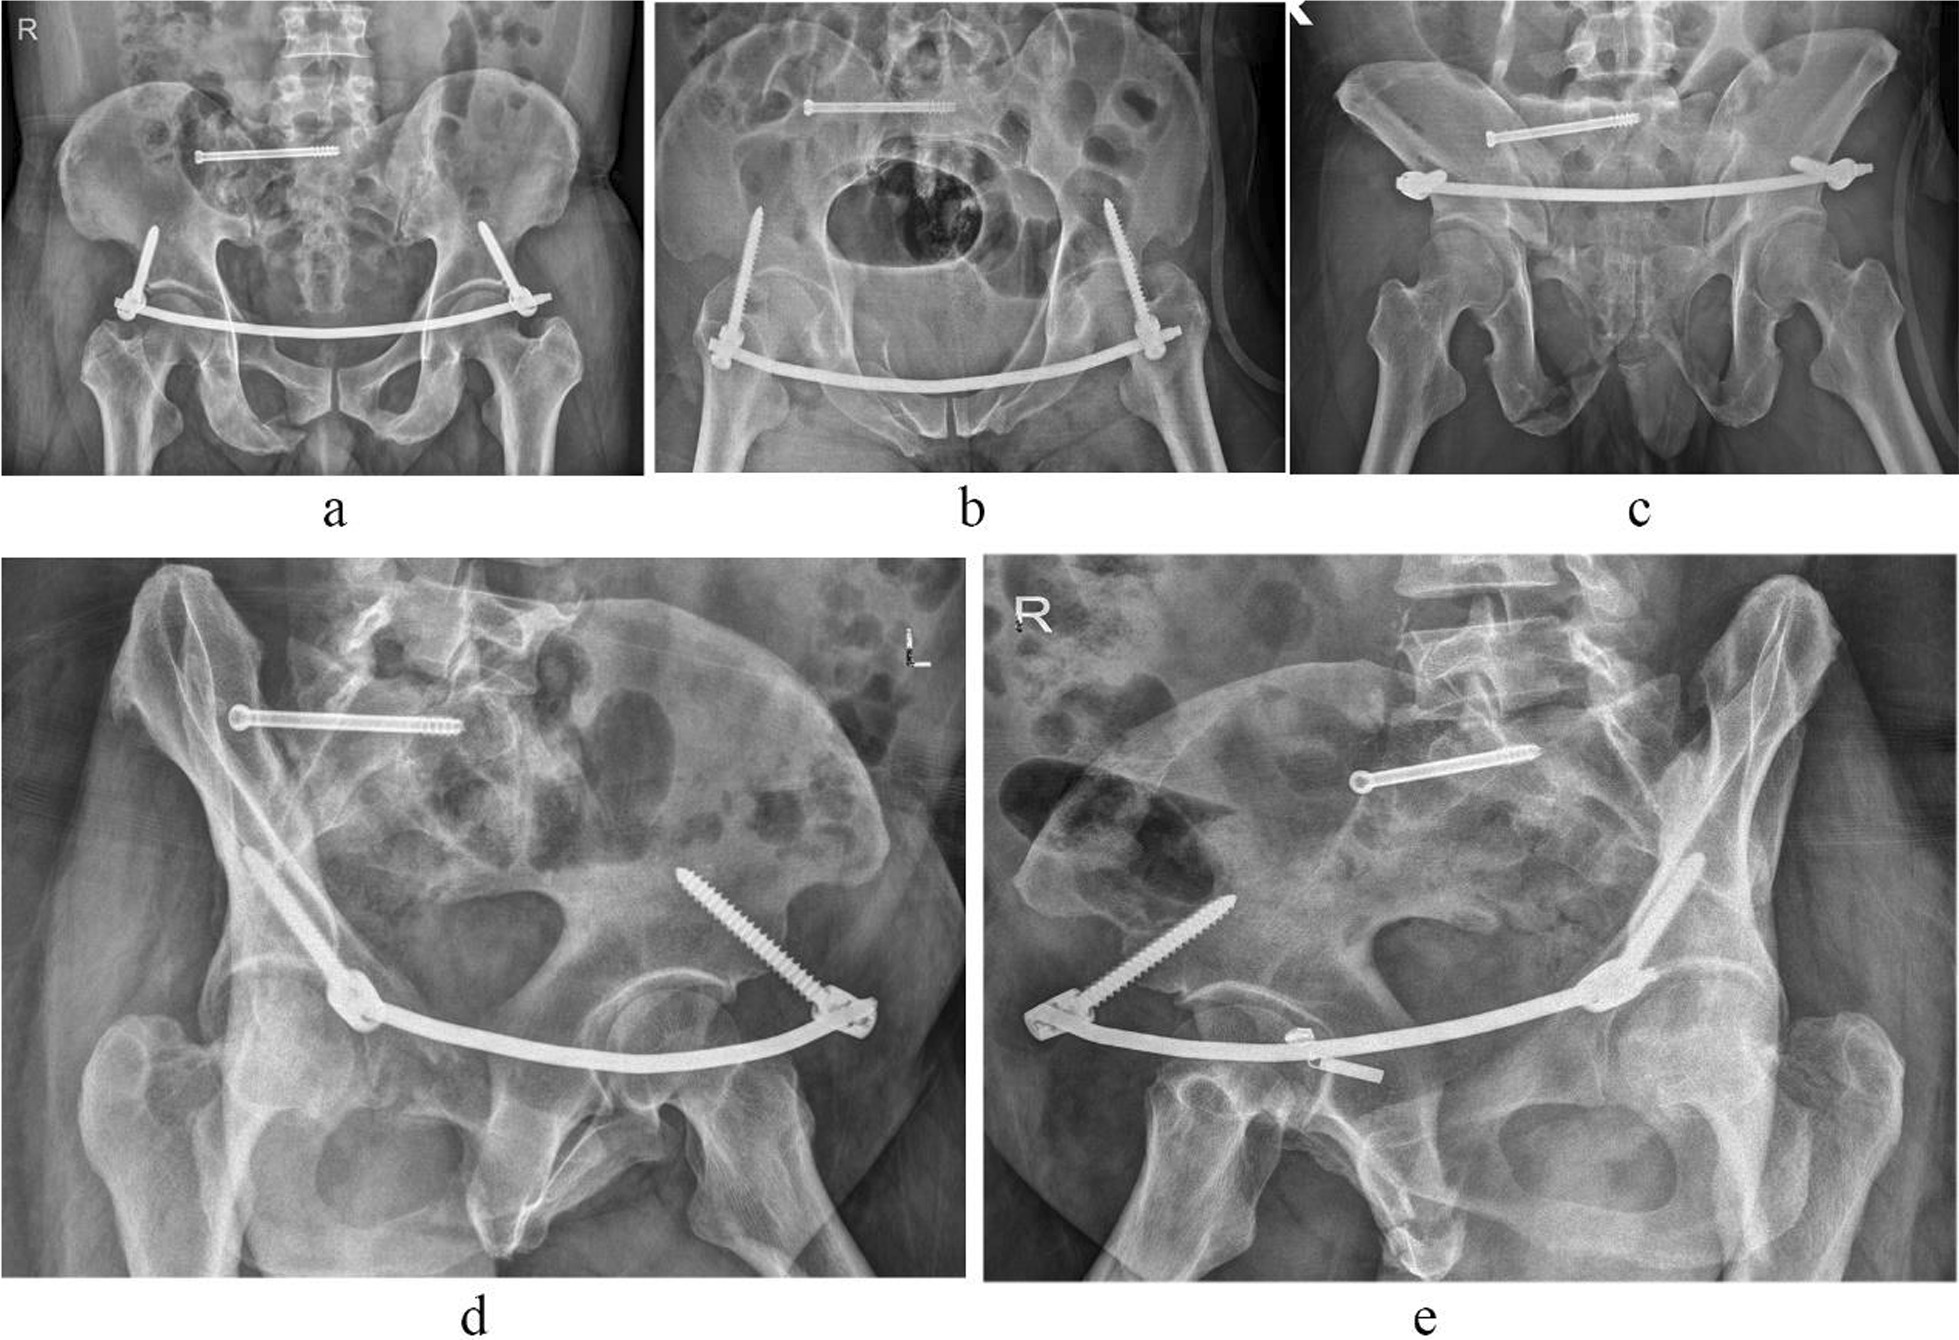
Postoperative images of case 1. (a Pelvic anteroposterior X-ray image, b pelvic inlet X-ray image, c pelvic outlet X-ray image, d obturator oblique position X-ray image, e iliac oblique position X-ray image.)
